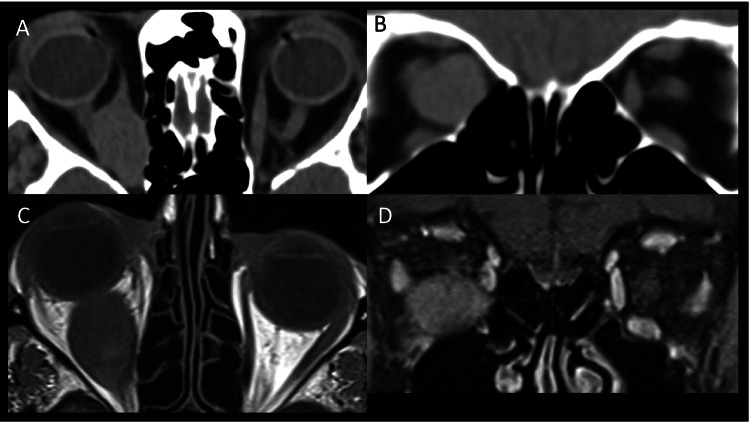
Intramuscular lymphoma. Case 1—axial (A) and coronal (B) CT scan showing significant enlargement of the right medial rectus muscle without involving the anterior tendon and causing optic nerve displacement. Case 2—axial T1-weighted MRI (C) shows isointense enlargement of the right inferior rectus muscle and right proptosis. Coronal fat-suppressed contrast-enhanced T1-weighted MRI (D) demonstrates heterogenous enlargement of the right inferior rectus muscle
